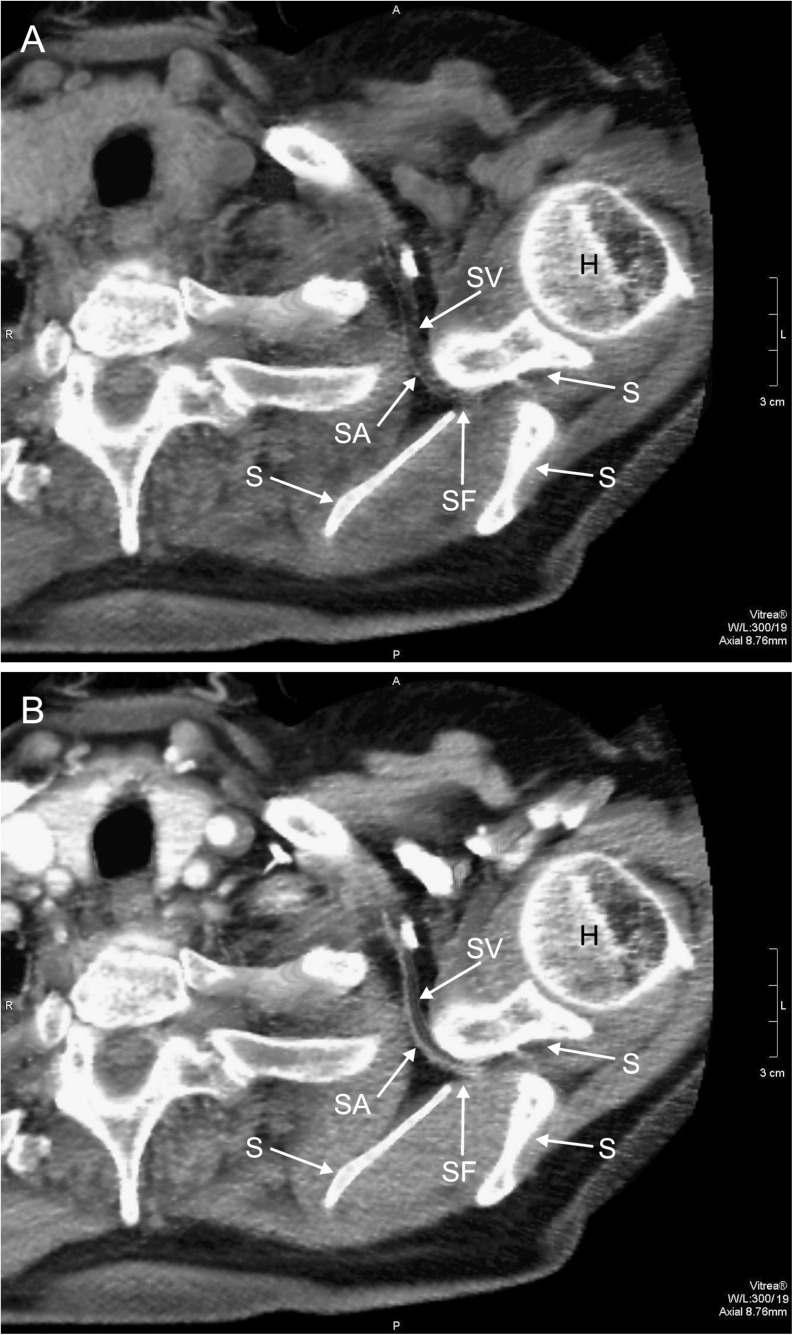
Dual-phase helical CT, transverse scan on the level of the superior suprascapular foramen: *A* without contrast material, *B* after injection of contrast medium. *H* humerus, *SF* superior suprascapular foramen, *SA* suprascapular artery, *SV* suprascapular vein, *S* scapula
